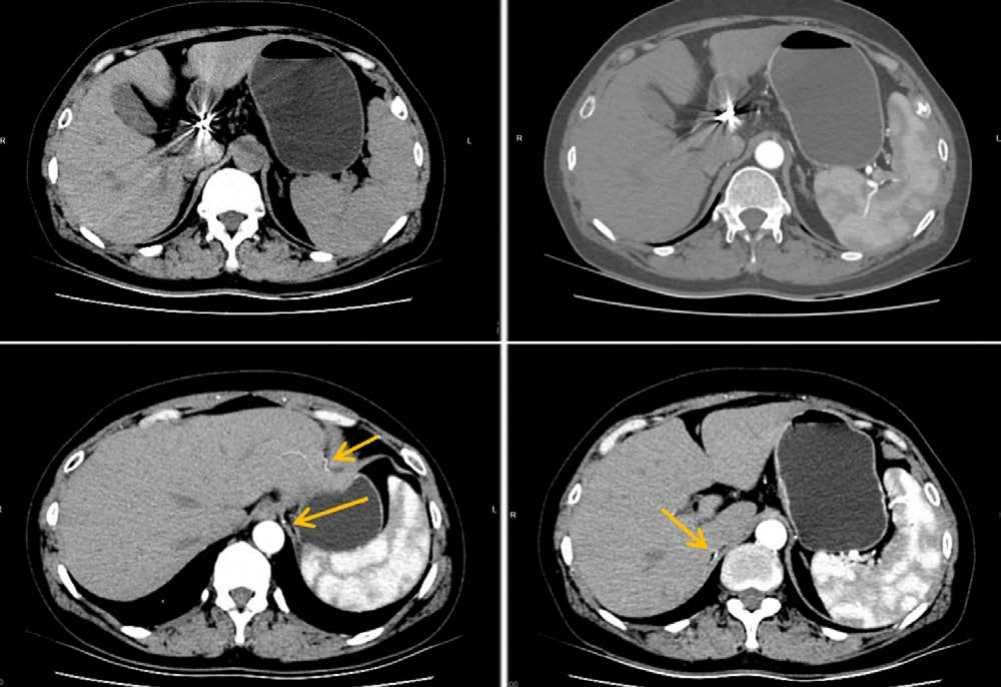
Enhanced CT – 1 mo after embolization: intrahepatic arterial blood flow is homogeneous throughout the liver.
